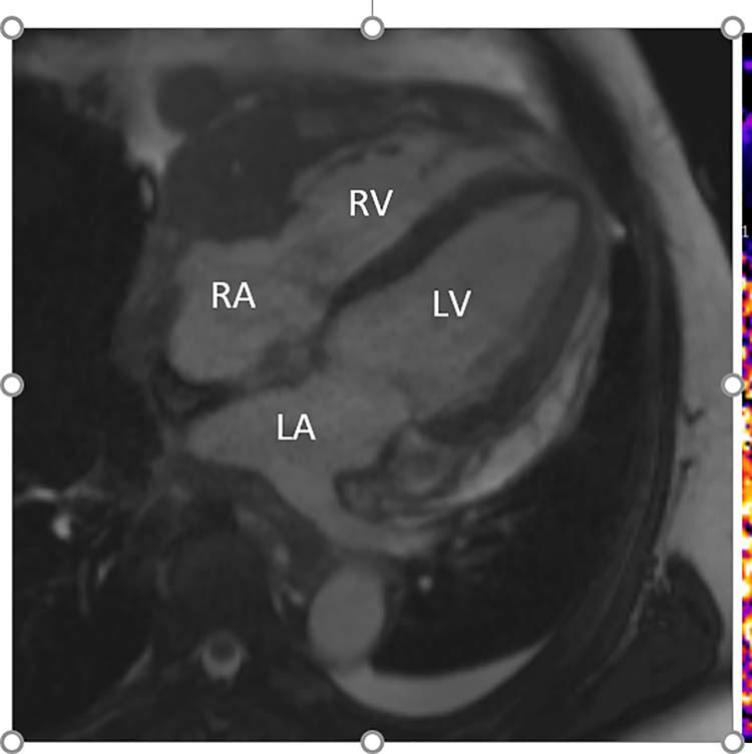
Cardiac MRI (transverse plane) demonstrating an enhancing lesion surrounding the chambers of the heart.
